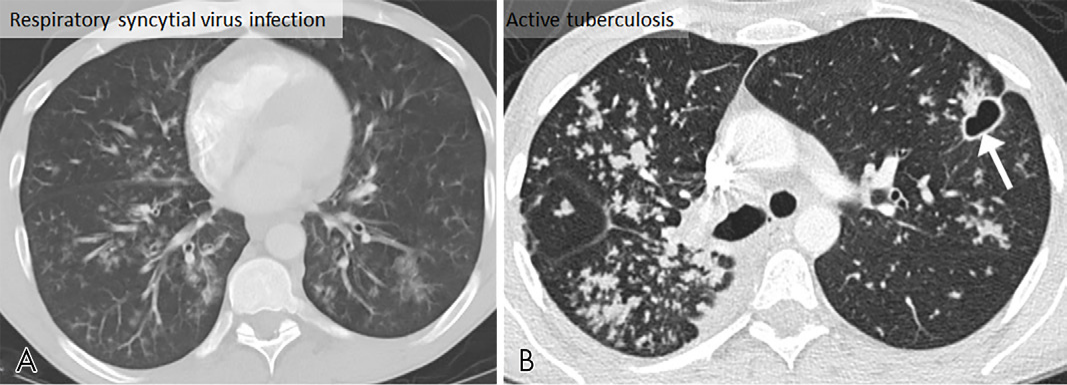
Atypical CT imaging features for COVID-19. Axial CT images from two different patients showing tree-in-bud opacities and centrilobular nodules, caused by respiratory syncytial virus (*A*) and active tuberculosis (*B*). A small cavity (arrow) is also present in (*B*) Routine screening CT for diagnosis or exclusion of COVID-19 is currently not recommended by most professional organizations or the U.S. Centers for Disease Control and Prevention.
